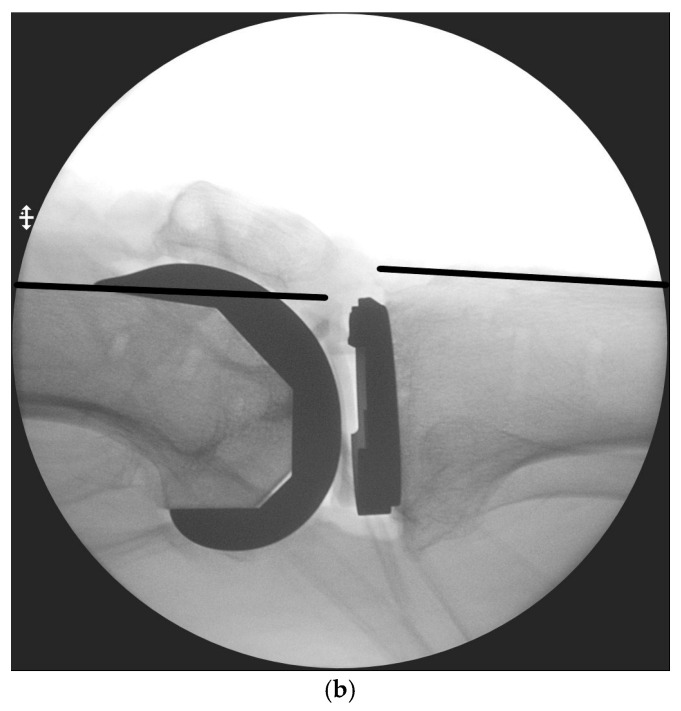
(a) Intraperative C-arm radiographs obtained with the final insert and C-arm beam aligned parallel to the tibial plateau. (b) Lateral fluoroscopic image to assess sagittal alignment intraoperatively. The angle between the anterior cortex of the distal femur and the anterior cortex of the proximal tibia is measured.
